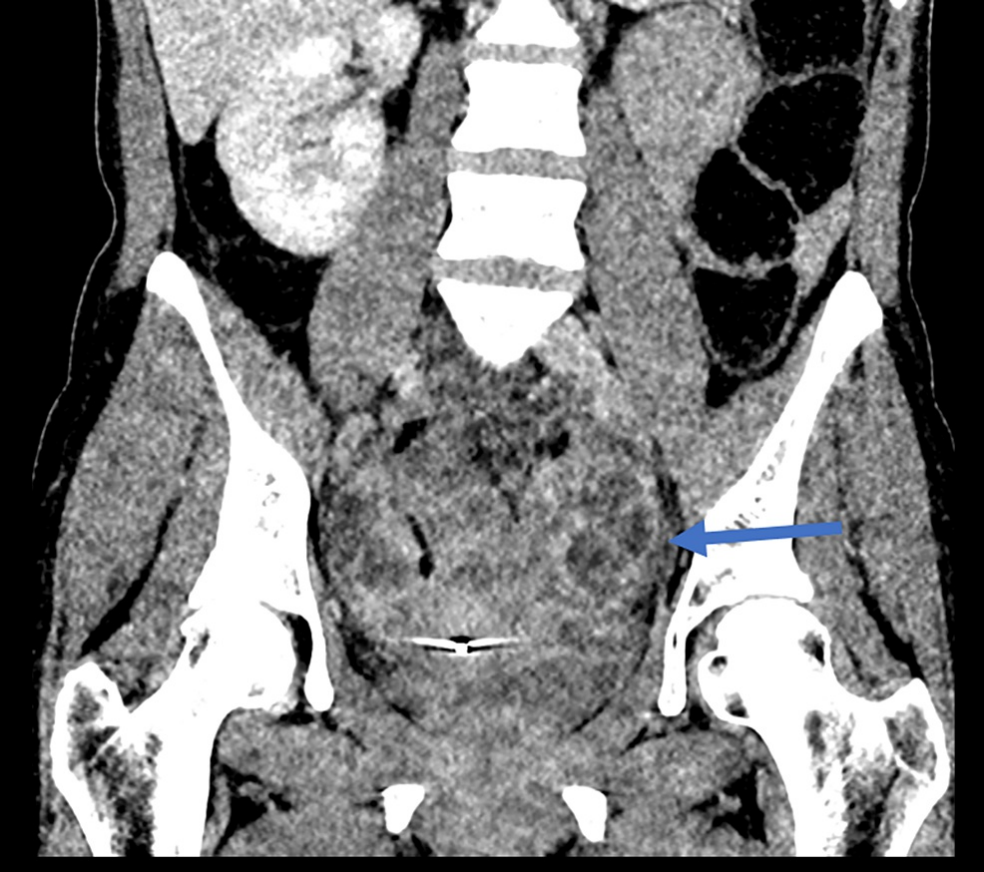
Coronal view of CT of the abdomen and pelvis. Blue arrow showing coronal view of abscess.
